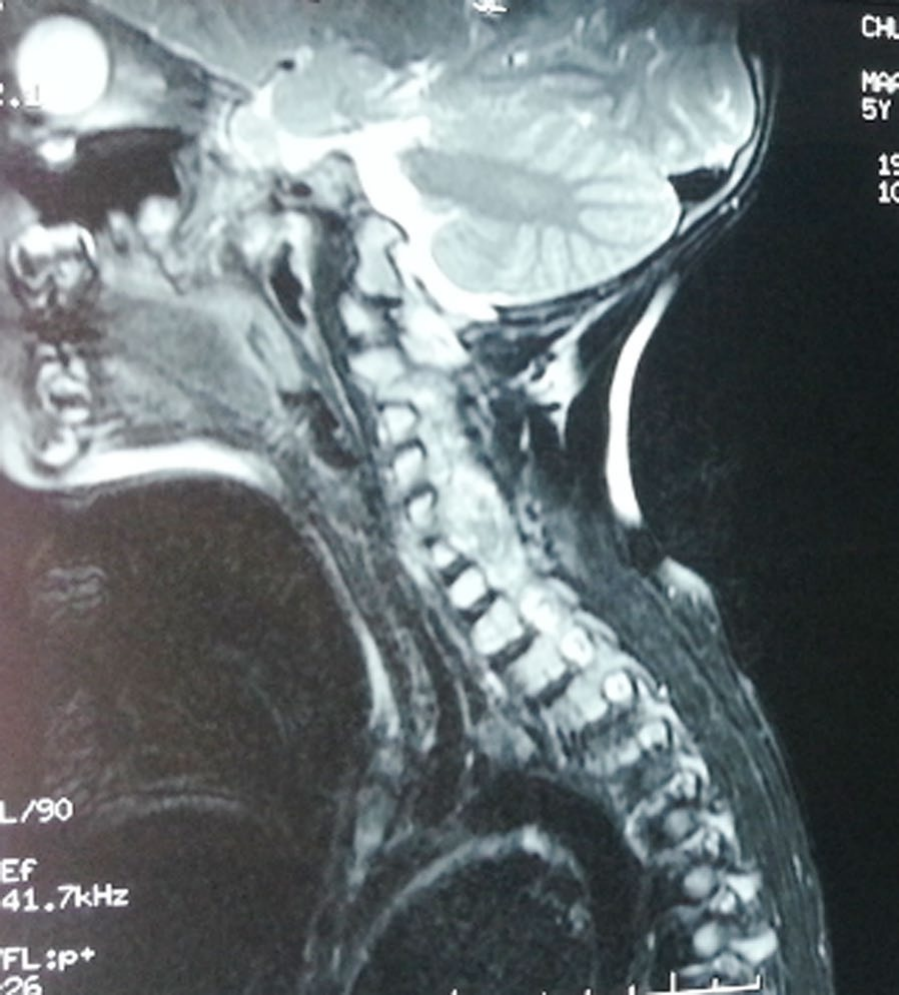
Sagittal T2 MRI showing an extradural mass observed at C1–C6 level
